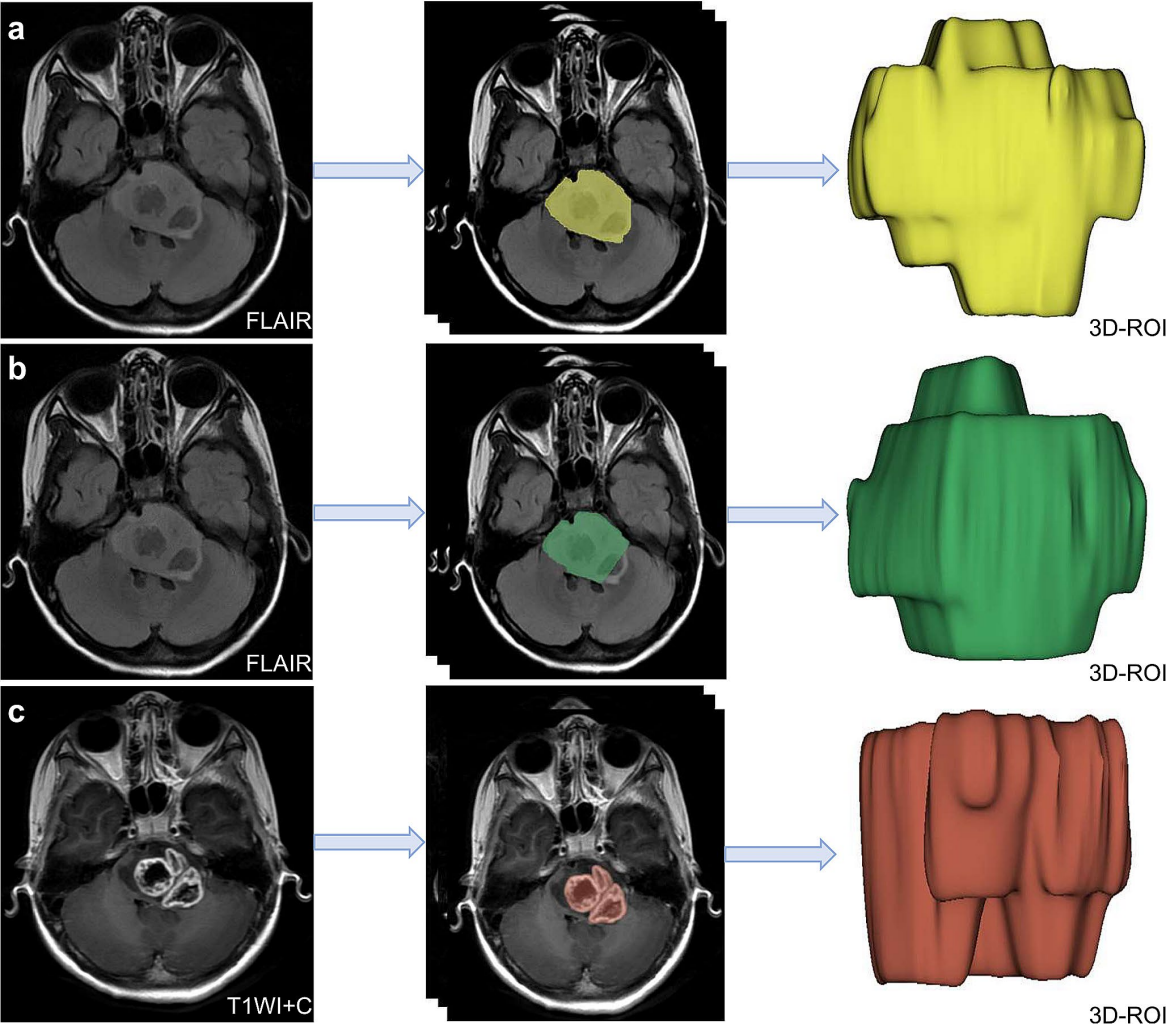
Volume measurements. (a-b). Axial T2-FLAIR images used to measure total tumor volume (yellow) and pontine tumor volume (green) in DIPG. (c). Axial T1WI + C used to measure ring enhancement volume (red) in DIPG. Extrapontine tumor volume was defined as total tumor volume minus pontine tumor volume DIPG, diffuse intrinsic pontine glioma; FLAIR, fluid-attenuated inversion recovery; T1WI + C, contrast-enhanced T1WI; 3D-ROI, three-dimensional region of interest
